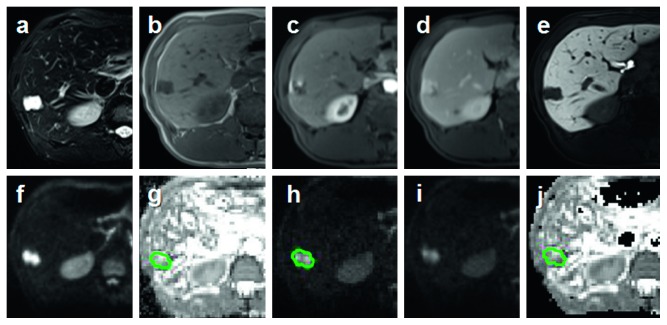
ROI was drawn on the level of maximum transactional diameter of hemangioma (green circle). (a, b) Axial fat suppress T2WI and T1WI. (c–e) Dynamic enhancement imaging included artery phase, portal phase, and hepatobiliary phase (HBP). (f, g) Axial liver DWI (*b* = 800 s/mm^2^) and ADC maps. (h–j) Axial liver DKI (*b* = 2000 s/mm^2^) and DKI-derived parameters *K* and *D* maps.
